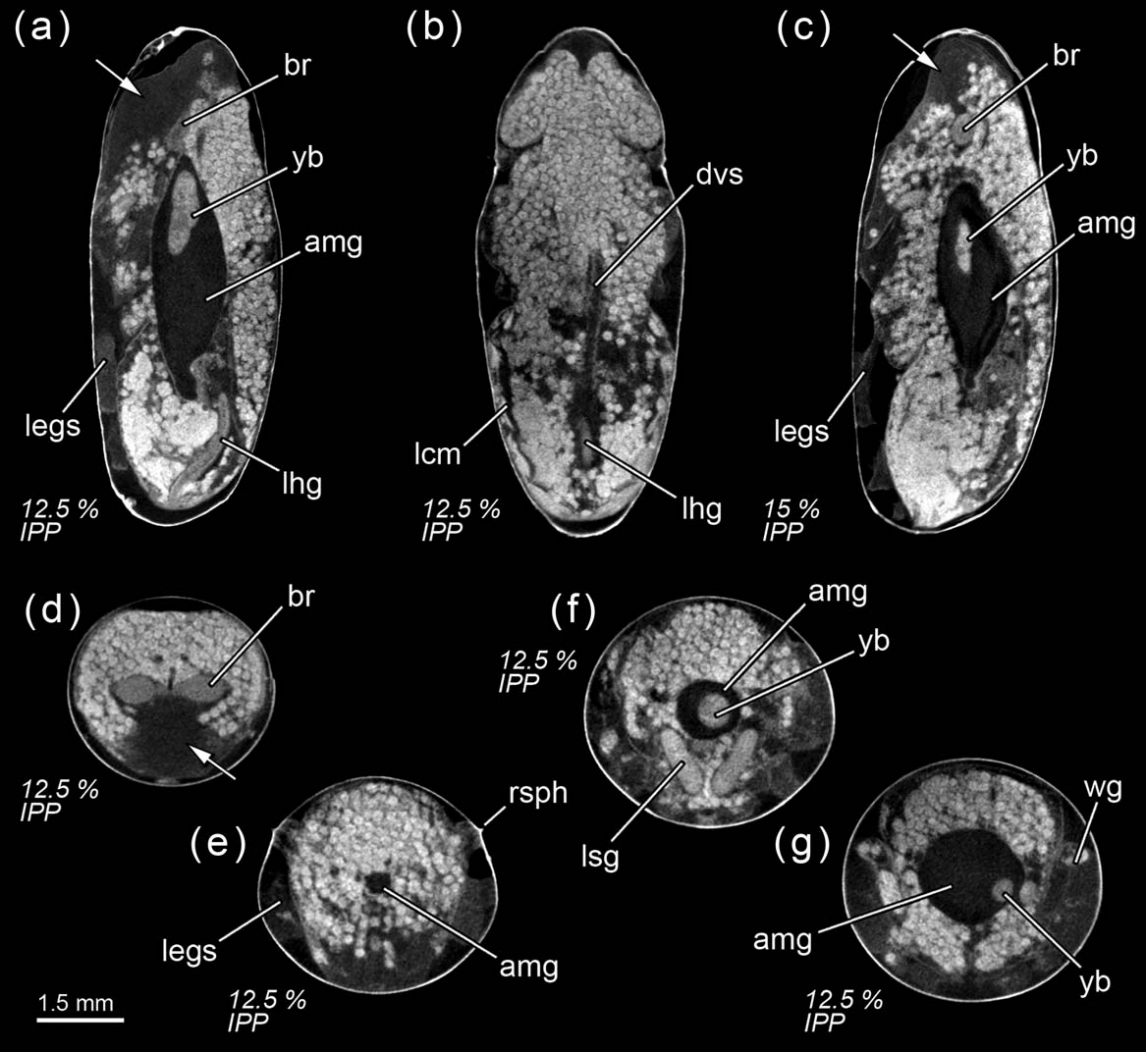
Calliphora vicina*, micro‐CT‐based virtual sections of puparia at different times after pupariation (AP), reared at 24°C. The corresponding percentage of time of the total intra‐puparial period (IPP) is given in brackets after each time. (a) 30 hr AP (12.5% IPP), medial sagittal section. (b) 30 hr AP (12.5% IPP), dorsal horizontal section. (c) 36 hr AP (15% IPP), medial sagittal section. (d) 30 hr AP (12.5% IPP), medial cross section of the head. (e) 30 hr AP (12.5% IPP), anterior cross section of the thorax. (f) 30 hr AP (12.5% IPP), antero‐medial cross section of the thorax. (g) 30 hr AP (12.5% IPP), medial cross section of the thorax. amg, adult midgut; br, brain; legs, legs; lhg, larval hindgut; lhm, larval hypodermal muscles; rsph, respiratory horns; wg, wings; yb, yellow body. Arrows indicate the hyaline regions on the head where the fat bodies have still not migrated
